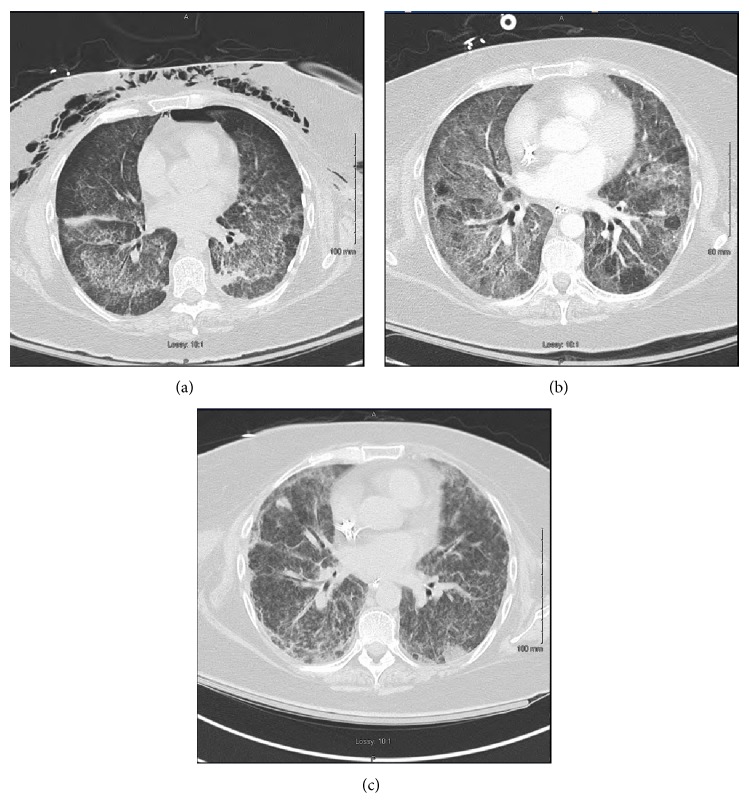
(a) CT of the chest without contrast on Day 10 showing marked improvement in airspace opacities, (b) Day 30 showing increase in diffuse ground glass opacities with associated reticular abnormality, (c) and Day 40 showing further increase in ground glass opacities with evidence of interstitial fibrosis.
